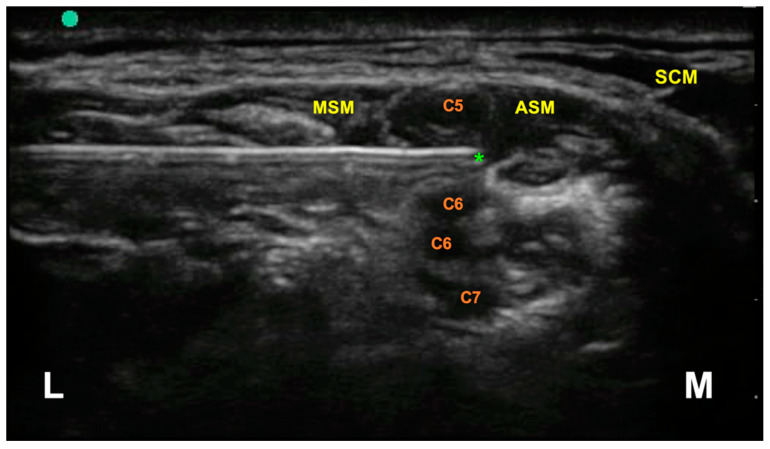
Interscalene brachial plexus, ultrasound anatomy. The asterisk indicates the target of the needle tip during the block, located between the C5 and C6 nerve roots. MSM = medial scalene muscle; ASM = anterior scalene muscle; SCM = sternocleidomastoid muscle; L = Lateral; M = Medial.
